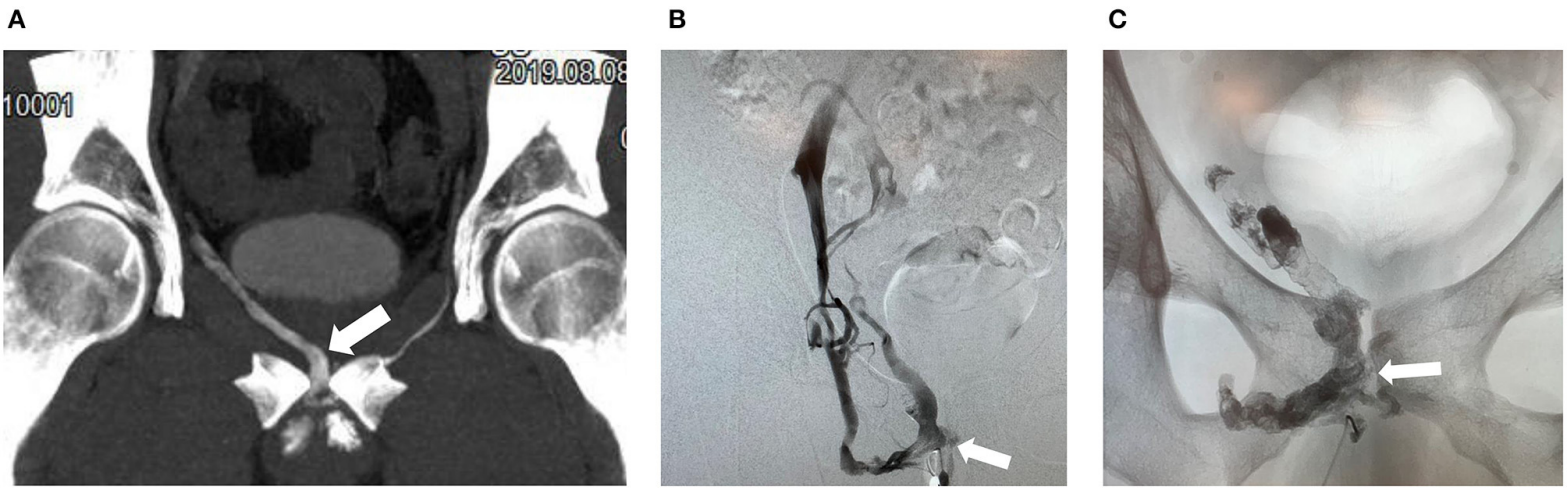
50-year old man with erectile dysfunction due to veno-occlusive disease. (A) Contrast enhanced CT cavernosography (coronal maximum intensity projection) demonstrates right sided venous leak via periprostatic veins (arrow). (B) Cavernosography with access via the deep dorsal vein and injection of contrast medium demonstrates right sided venous leaks predominatentely via periprostatic veins (arrow). (C) Post embolization of periprostatic veins (arrow) using N-butyl-2-cyanoacrylate mixed with Lipiodol demonstrating radiopaque intravenous embolization material (arrow).
